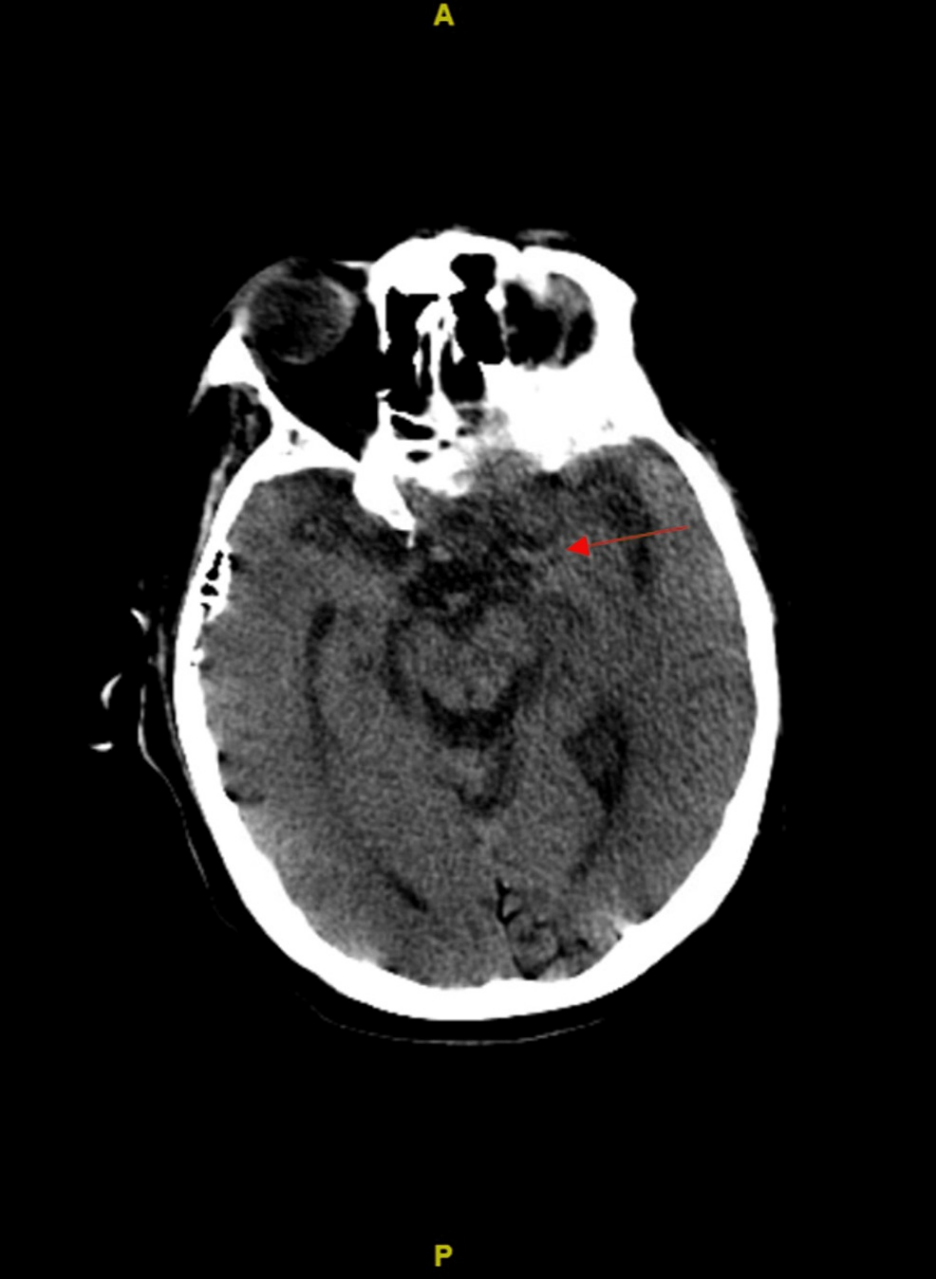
Non-contrast CT head axial image. Initial CT head without evidence of emboli in the middle cerebral artery highlighted by the red arrow.
